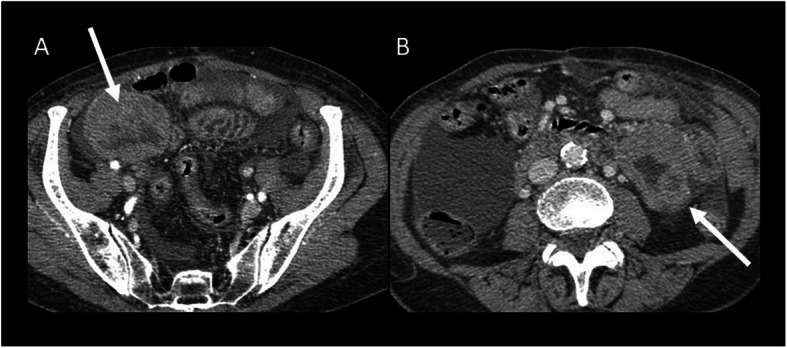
Ileal and digiunal GIST. CT shows two masses (arrow) with an extensive mesenteric component characterized by central necrosis, which manifest as focal areas of hypoattenuation
